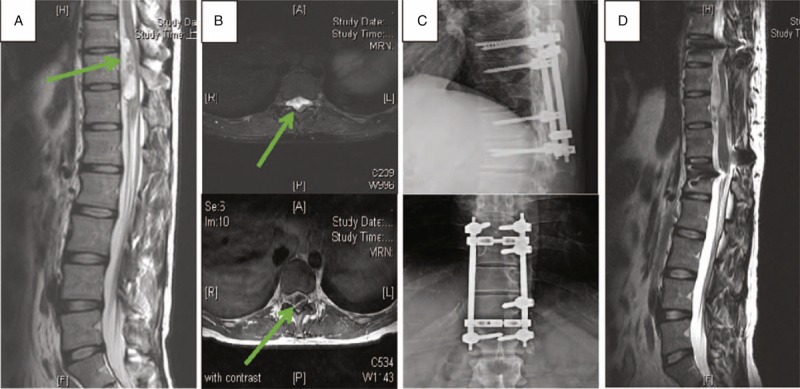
Radiological studies of Case 1. The green arrows mark the position of tumor. (A) Preoperative sagittal T2-weighted imaging of T-spine magnetic resonance imaging (MRI). (B) Preoperative axial imaging of T-spine MRI. Upper image is T2 weighted and lower image is Gd-enhanced T1 weighted. (C) Postoperative T-spine X-ray anteroposterior and lateral view. (D) Sagittal T2-weighted imaging of T-spine MRI at 2 y postsurgery.
